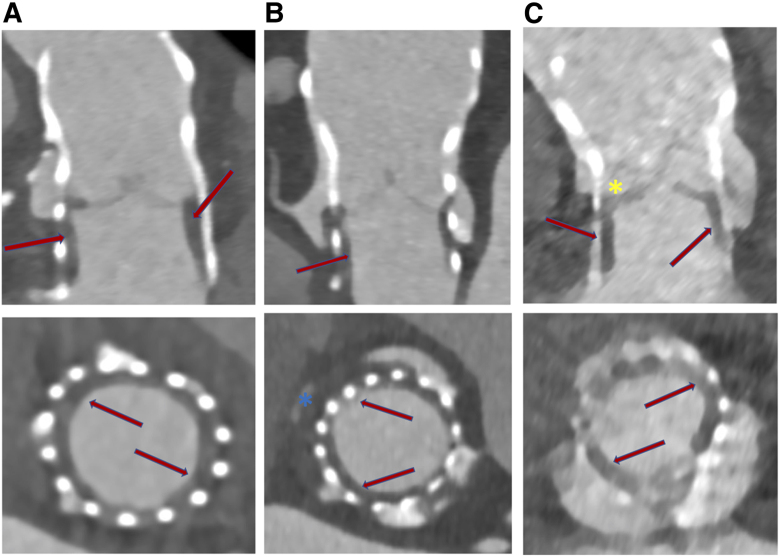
(*A-C*) Subvalvular thrombosis visualised with multi-detector computed tomography in long and short axes (red arrows). Asterisk in the (*B*) panel indicates association with anatomic sinus thrombosis. Asterisk in the (*C*) panel indicates concomitant leaflet thrombosis.
